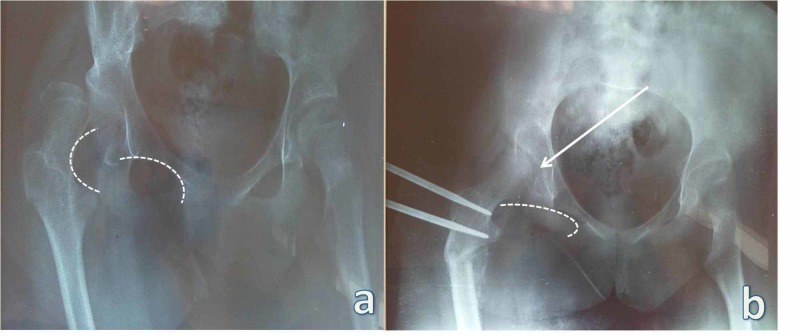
Restoration of femoral head-to-acetabulum congruity (arrow) a: X-ray demonstrating Shenton's line (interrupted curve) preoperatively; b: X-ray demonstrating Shenton's line (interrupted curve) postoperatively
